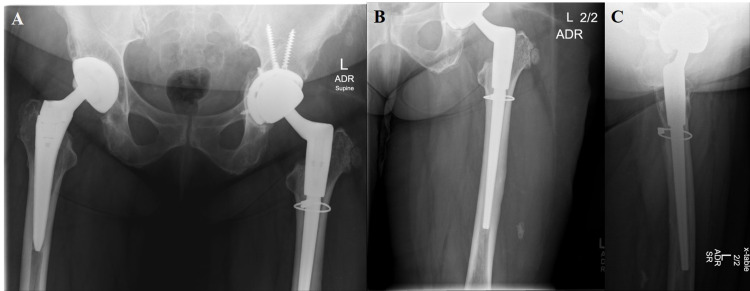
One-year postoperative radiographs [anterior-posterior pelvis (A), anterior-posterior left hip (B), lateral left hip (C)] after stage 2 of second revision using Stryker Res Mod system and cabling for femoral neck stress fracture with successful eradication of infection.
